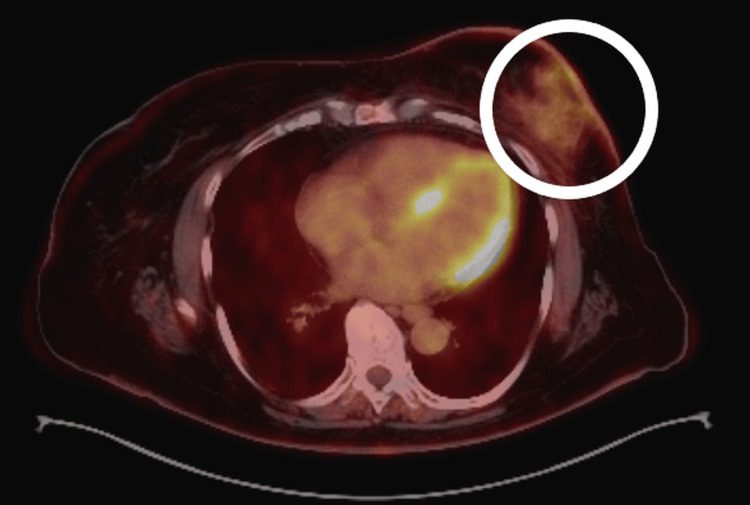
Axial PET/CT image (April 2023) demonstrating focal fluorodeoxyglucose uptake in the left breast/chest wall (circled), without evidence of distant metastatic disease.
